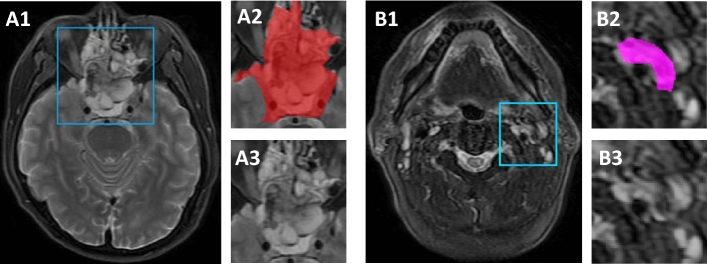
MRI examples of LA-NPC including tumors and lymph nodes. A1  Full-field MRI of tumor, A2  tumor with its mask, A3  MRI patch of tumor; B1  full-field MRI of lymph node, B2  lymph node with its mask, *B3*  MRI patch of lymph node
